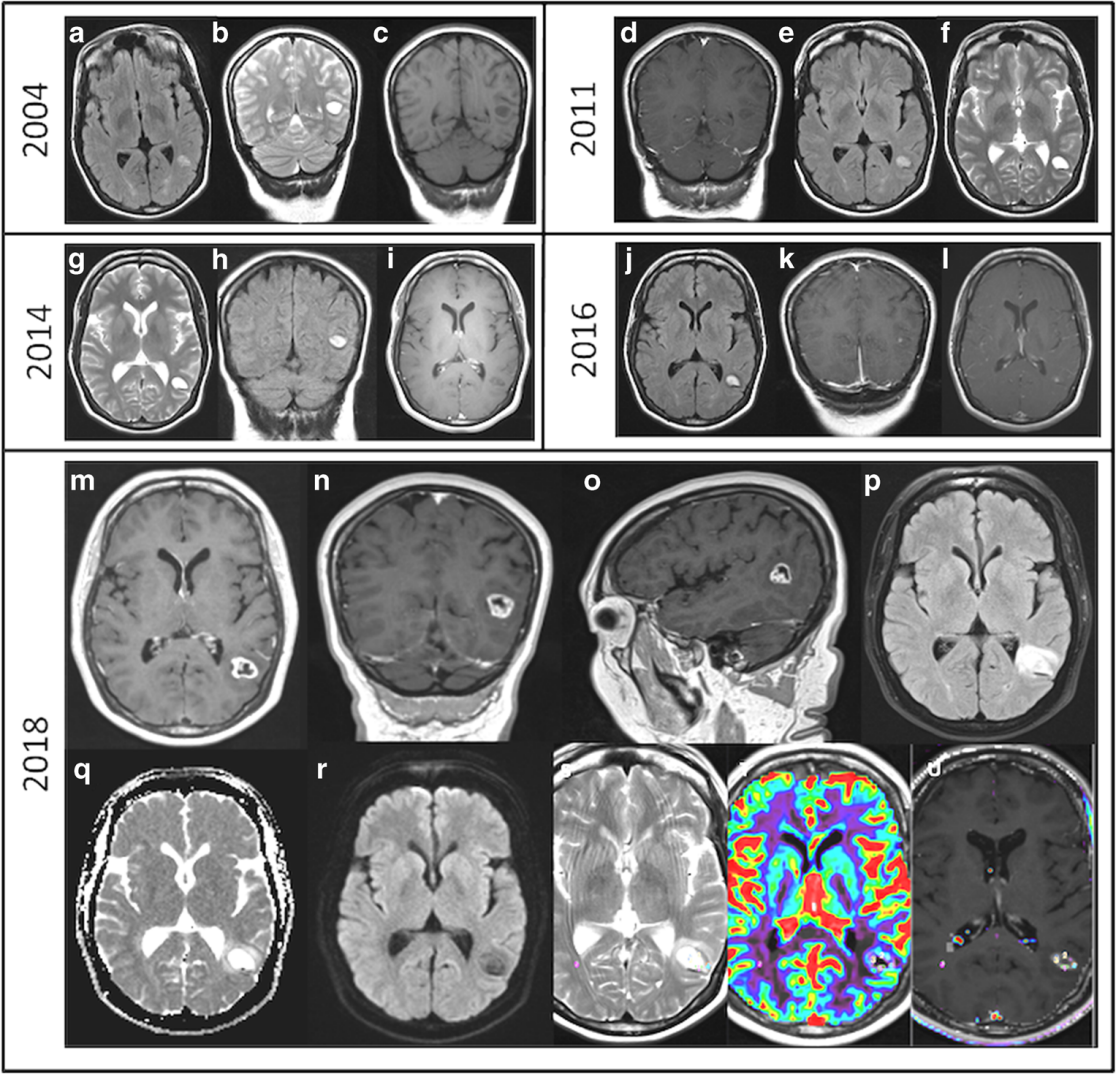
Evolution of the temporal-parietal-occipital lesion from 2004 to 2018. Slow growing lesion since 2004 with initial punctiform contrast uptake in 2016 that increased in size in 2018. 2004 (a, axial FLAIR; b, coronal T2; c, coronal T1); 2011 (d, coronal T1 GAD; e, axial FLAIR; f, axial T2); 2014 (g, axial T2; h, coronal FLAIR; i, axial T1 GAD); 2016 (j, axial FLAIR; k, coronal T1 GAD; l, axial T1 GAD); 2018 (m, axial T1 GAD; n, coronal T1 GAD; o, sagittal T1 GAD; p, axial FLAIR; q, ADC map; r, DWI; s, axial T2, t, u, CBV)
